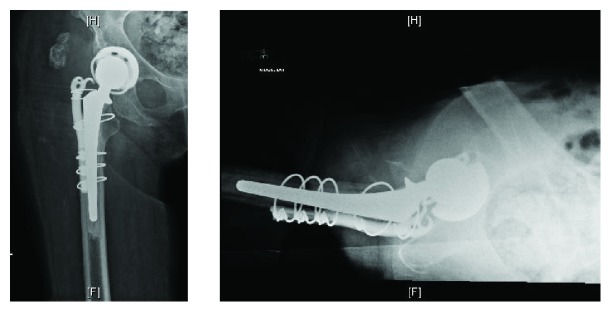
Anteroposterior (AP) and lateral right hip radiographs, seven months status post revision total hip arthroplasty with greater trochanter escape from hook plate.
